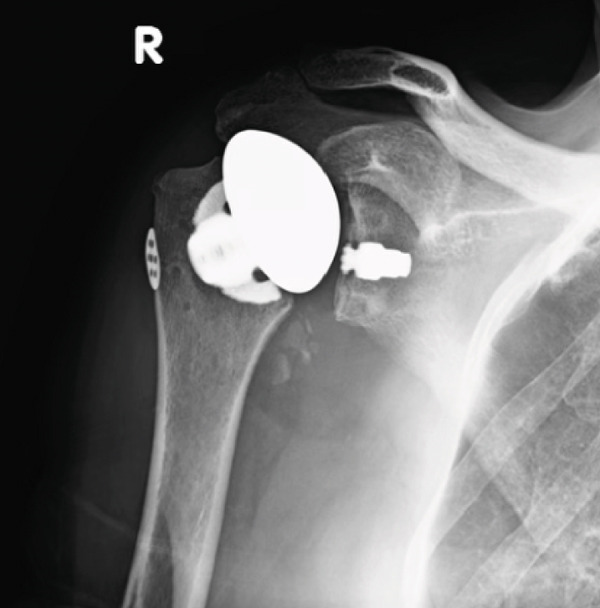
Shoulder radiograph demonstrating a stemless anatomic TSA at first postoperative visit (August 17, 2022).
